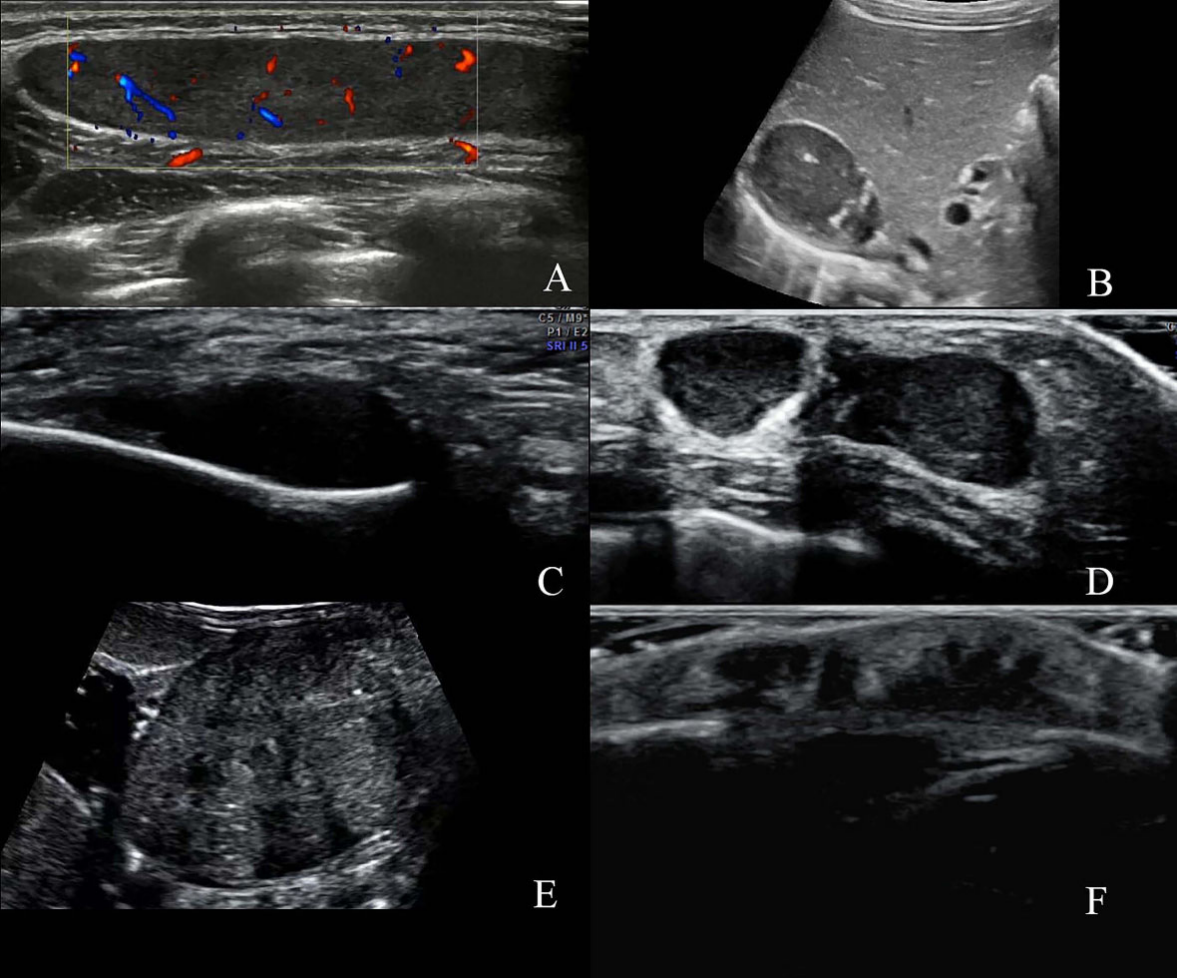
Superficial soft-tissue metastasis of a neuroblastoma. A, Female, 3y2m, right back mass for two years. A hypoechoic mass was observed between the subcutaneous fat layer and innermost intercostal area in the right back. This lump was well circumscribed with internal homogeneous echogenicity without patchy hyperechoic foci. Color Doppler flow imaging showed blood flow signals as dot strips. B, The adrenal area on the right side revealed a hypoechoic mass of 4.3×4.0×2.8 cm. The internal echo is hypoechoic, with hyperechoic foci. C, Female aged two months had numerous lumps in the whole body for one month. The front of the left ear showed a hypoechoic mass with a clear border and a homogeneous echogenic mass. D, The left chest wall indicated a hypoechoic lump with a clear border and heterogeneous internal echo. E, The left retroperitoneum presented a large substantial mass with a clear border and sparsely punctured calcification. The size was 8.0×5.5×4.5 cm. F, The top of the scalp showed an irregular hypoechoic mass with a clear border. It measured 1.0×1.0×0.6 cm. This lump had a heterogenic echo and did not indicate a prominent dark area or calcification. The blood flow signal in color Doppler flow imaging of the hypoechoic group was abundant.
